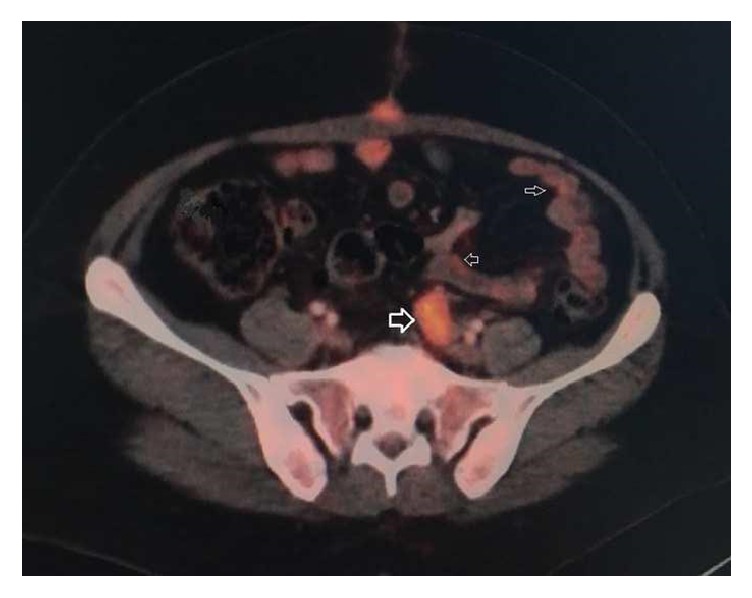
Abdominal positron emission tomographic (PET-CT) scan. Axial view shows variable-sized heterogeneous contrast-enhancing above rectosigmoid mesentery towards the left abdominal wall in pelvic floor (arrowheads). Arrow shows the lateral port site deposit.
